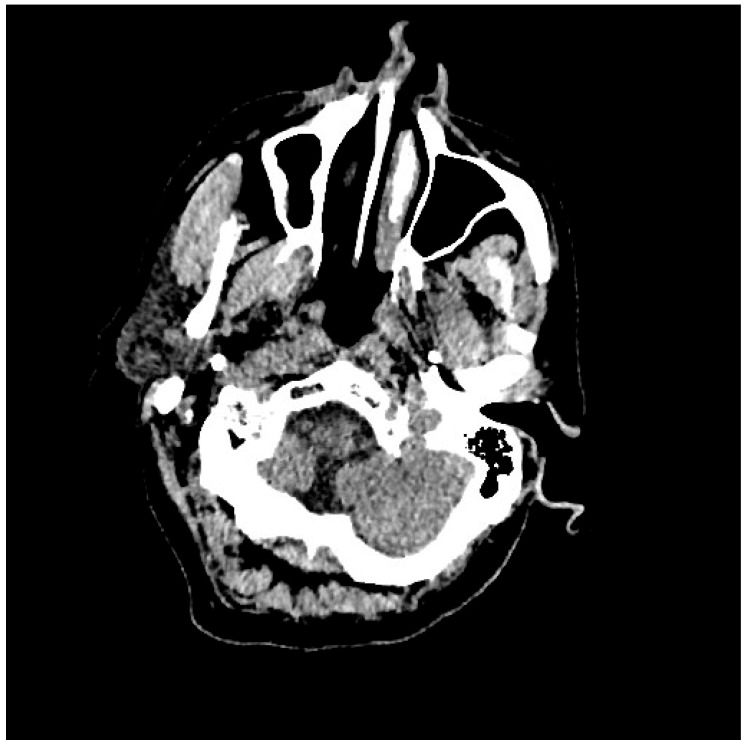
Evidence of ICH on brain CT scan (Table 1, patient 7).
